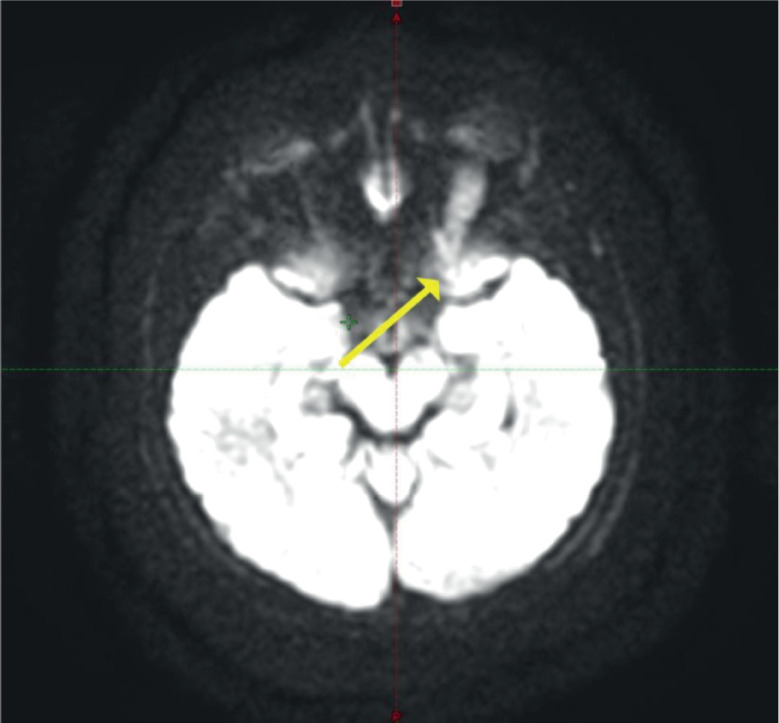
MRI of the brain and orbit showing an enlarged left optic nerve (yellow arrow).
